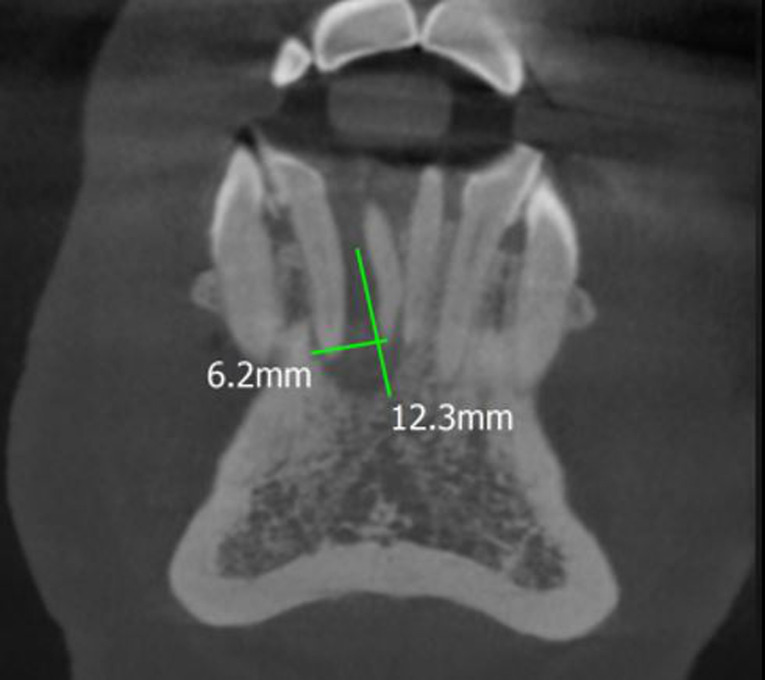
cone-beam computed tomography scan: peri-apical pathosis measuring about 12.3 x 6.2 mm with evidence of a bucco-lingual intra-bony osteolytic ill-defined area in relation to 41 and 42; presence of thin labial cortical plate was also noted
